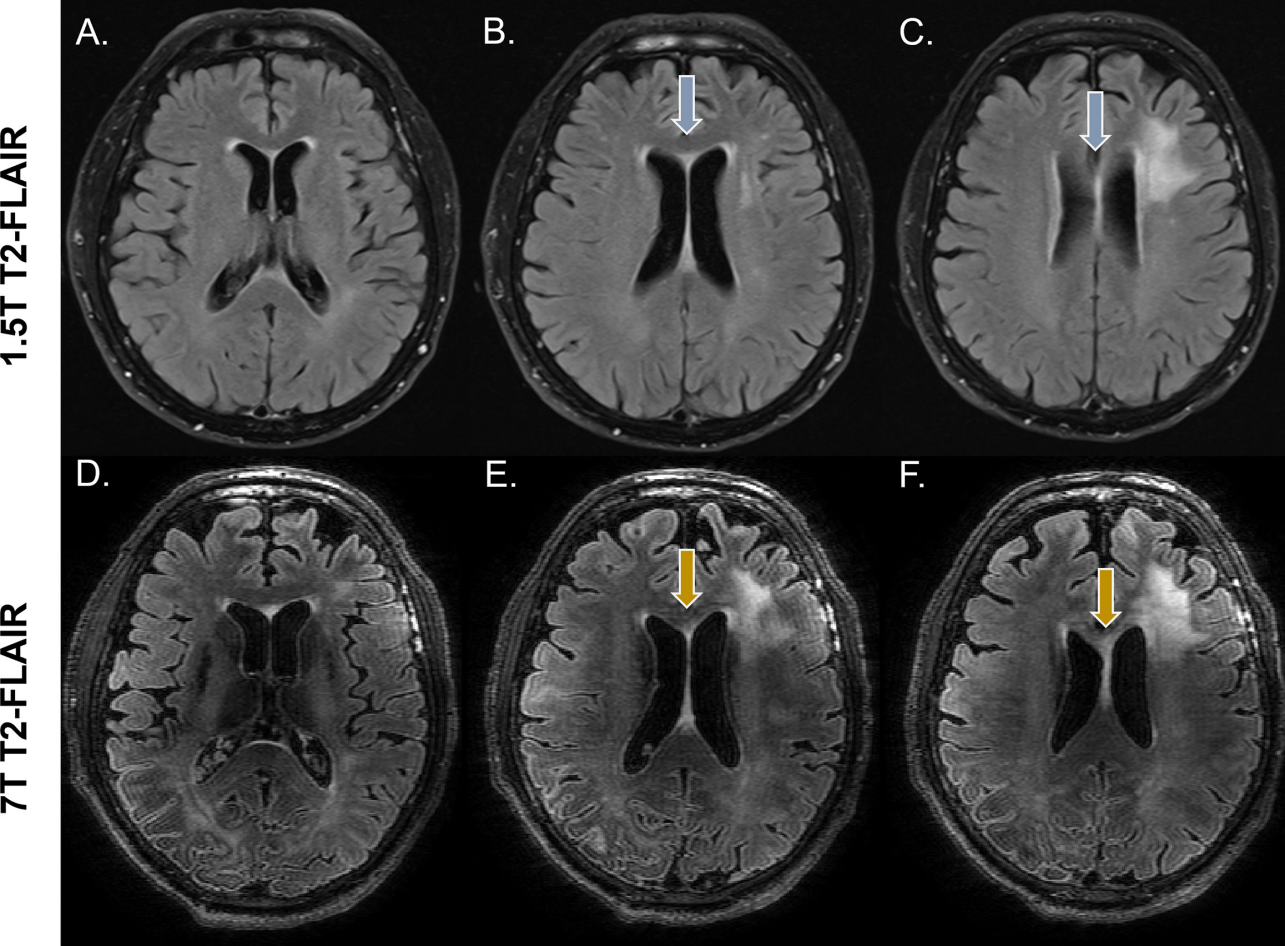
Visual assessment of tumor extension represented on T_2_‐FLAIR images in a patient with glioblastoma, IDH‐wild‐type, showing three representative slices of the tumor in A–C for the clinical 1.5 T T_2_‐FLAIR and in D‐F for the high‐quality 7 T T_2_‐FLAIR. In this case, the corpus callosum involvement is better observed in the high‐quality 7 T images. Especially, the connectivity between the T_2_ hyperintense area in the corpus callosum and the lesions can be more clearly followed on the high‐quality 7 T image compared with the clinical scan.
